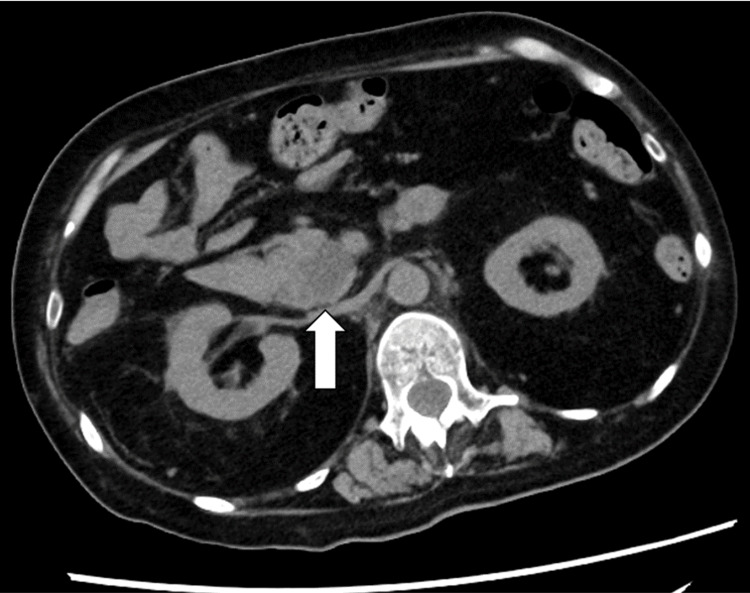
Findings of non-contrast computed tomography. A low-density area (arrow) was observed in the pancreatic head. Dilatation of the main pancreatic duct was not observed.
